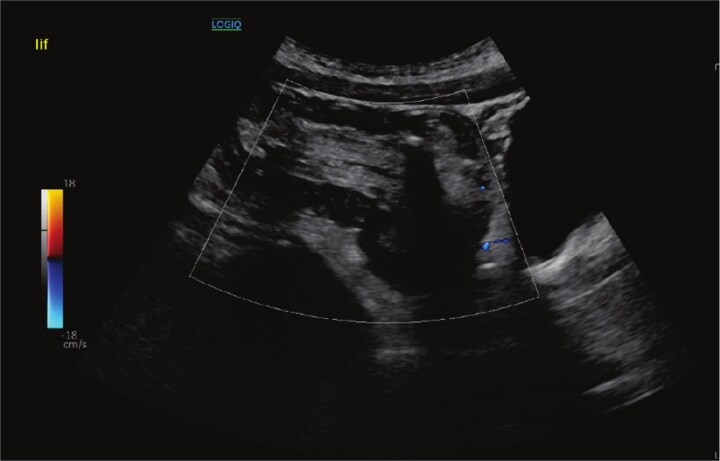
Abdominal ultrasound demonstrating a left iliac fossa mass suggestive of colo-colic intussusception.
